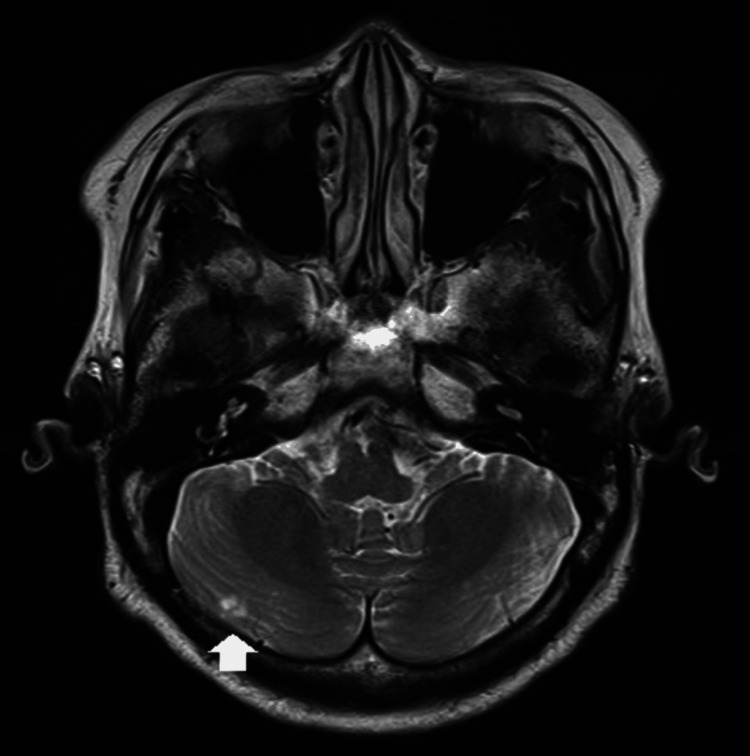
Head MRI T2-weighted image. A novel cerebral infarction was identified in the right cerebellum (arrow).
